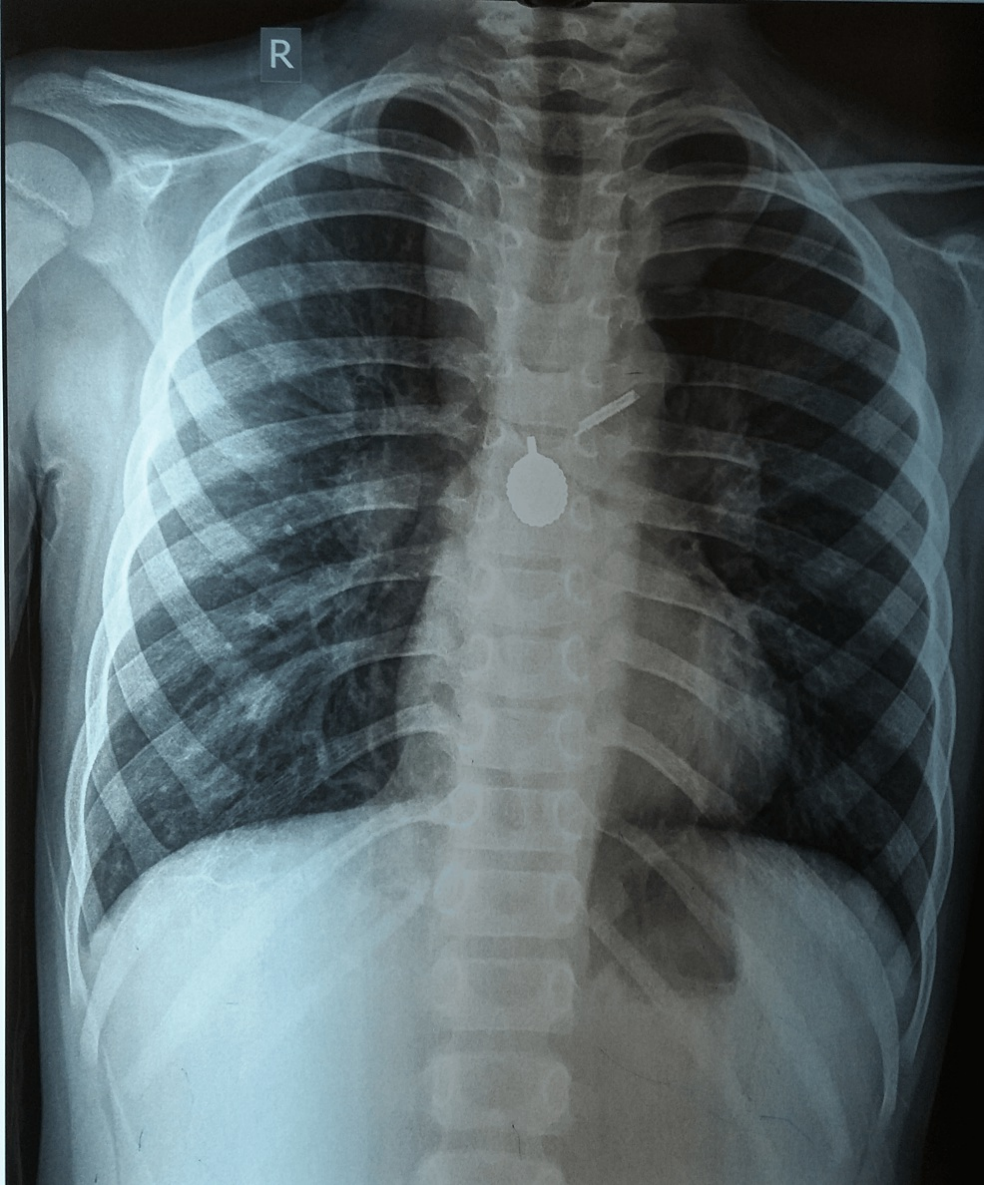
An unremarkable plain radiograph of the chest
